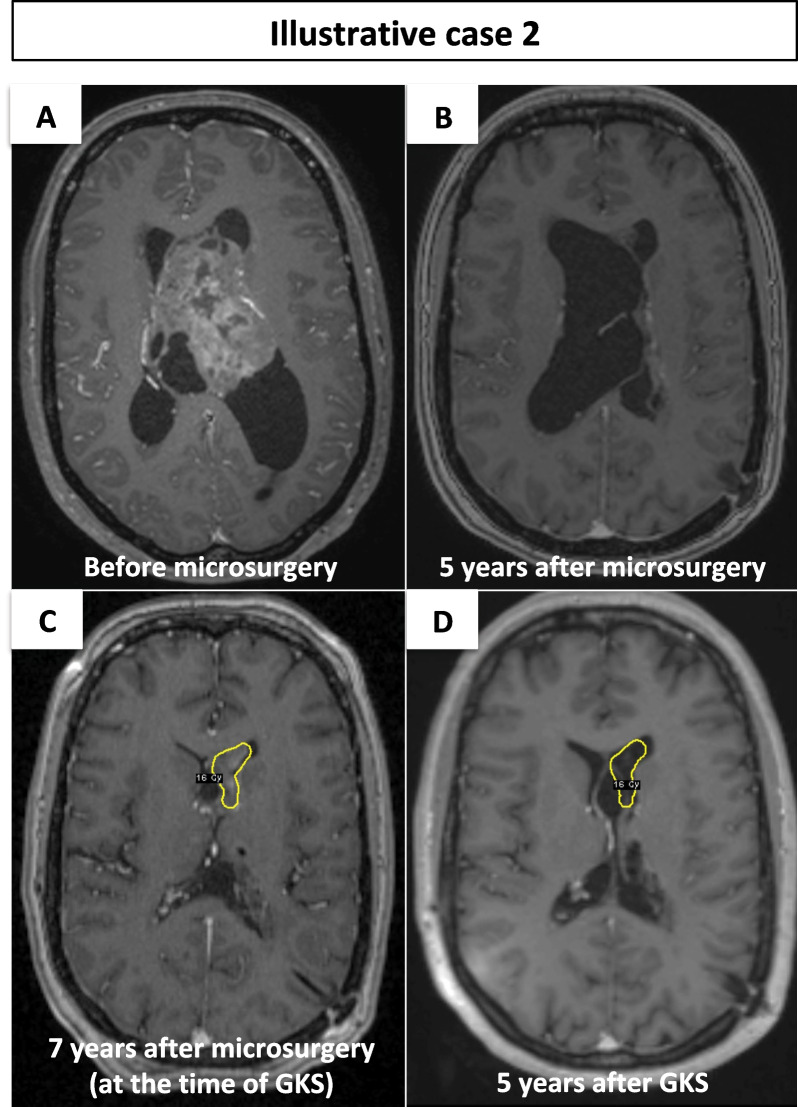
A Preoperative MRI; B 5 years postoperative MRI; C MRI at the time of GKS, showing the dosimetry, which is colored in yellow; D MRI 5 years after GKS, with superimposed dosimetry
